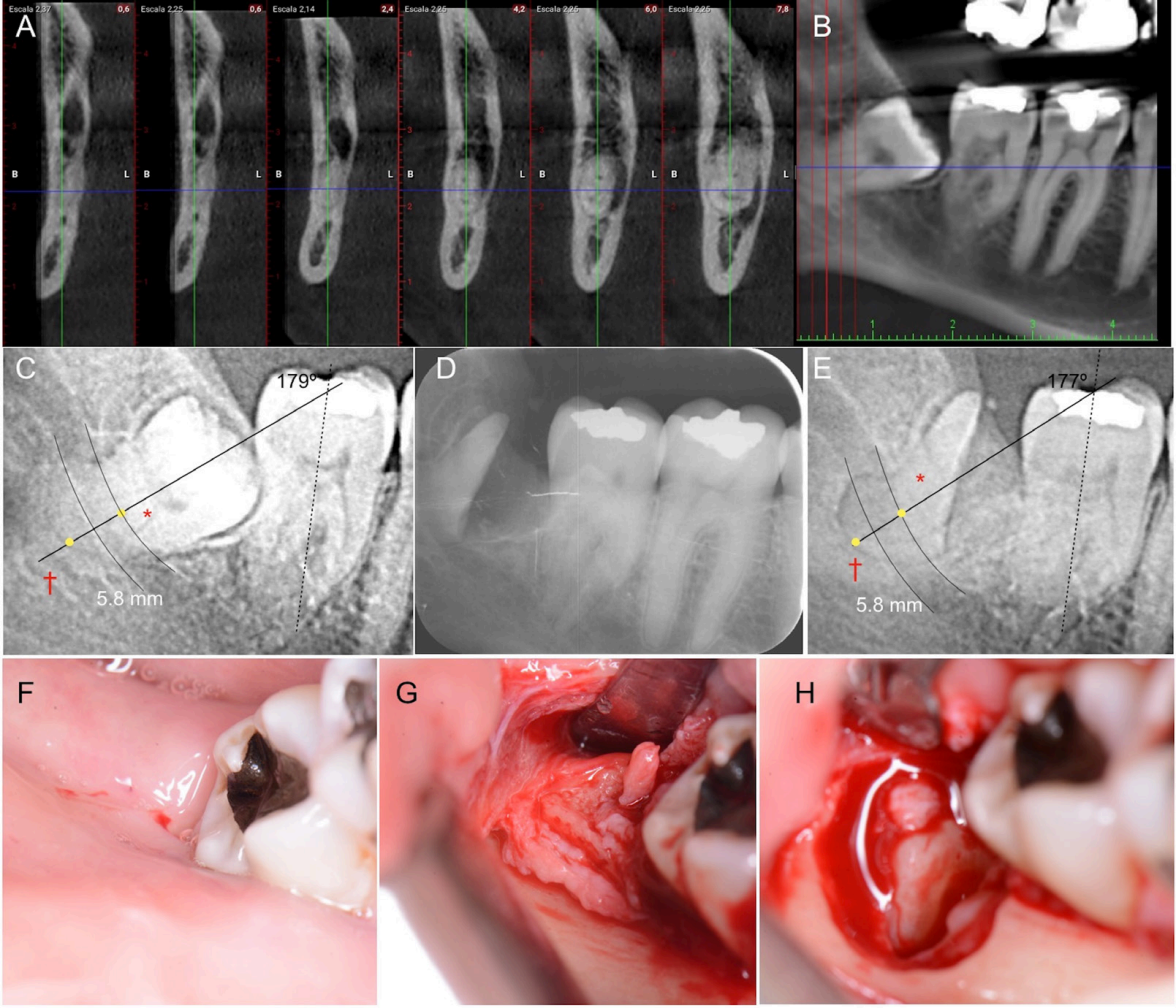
Case no. 2. Initial CBCT. A) Coronal sections separated at 1.80 mm; B) Sagittal section. C) Initial OPG, (D) periapical in the immediate postoperative period, (E) OPG at 13 months follow up (*Stable reference [upper part of the IAN canal] and, †most apical point of the radicular portion in the axial axis of the LTM). F) Initial intraoral clinical image of 4.8. covered by mucosa; G) Buccal mucoperiosteal flap elevation and protection of the lingual flap; H) Coronectomy of 4.8.
